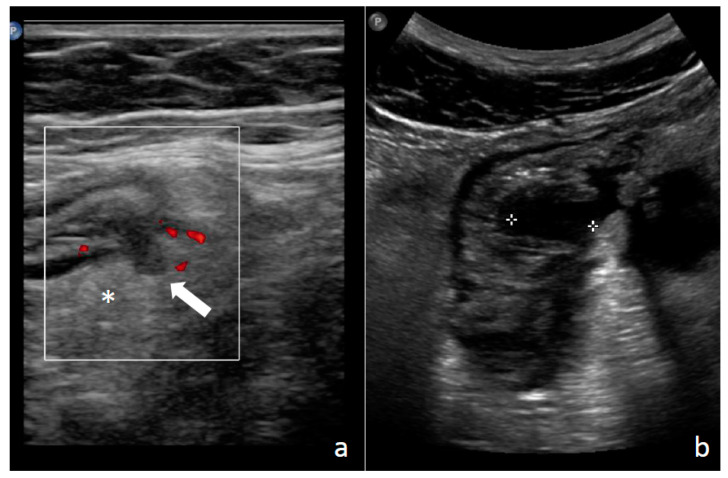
Diverticulitis on ultrasound imaging: (a) in the site of tenderness descending colon show a diverticulum as a bowel outpouching (arrow) surrounded by echogenic and non-compressible fat (*) with mild hypervascularization on power Doppler study; (b) a localized abscess appears as a hypoechoic fluid collection (between calipers) inside the thickened colonic wall.
